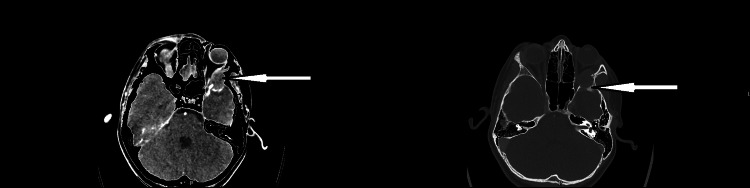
CT head axial section with soft tissue window (left side) and bone window (right side). The soft tissue window (left side) shows a collection at the left orbital wall, and the bone window (right side) shows bony destruction of the greater wing of the sphenoid bone.
